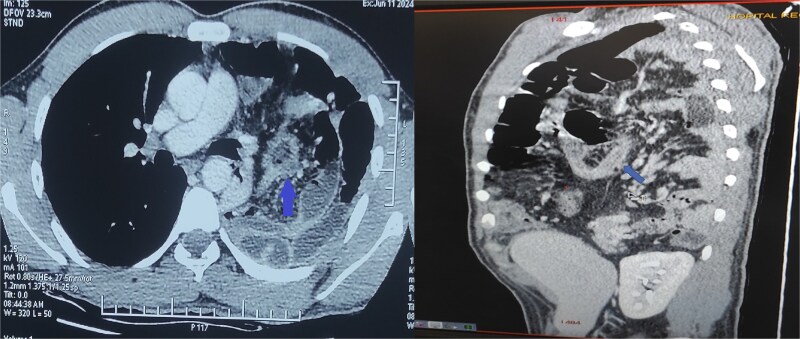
Contrast-enhanced CT of the chest and abdomen showing a left posterolateral diaphragmatic hernia with herniation of bowel and omentum into the thorax. The thickened appendix with surrounding fat stranding indicates acute appendicitis.
